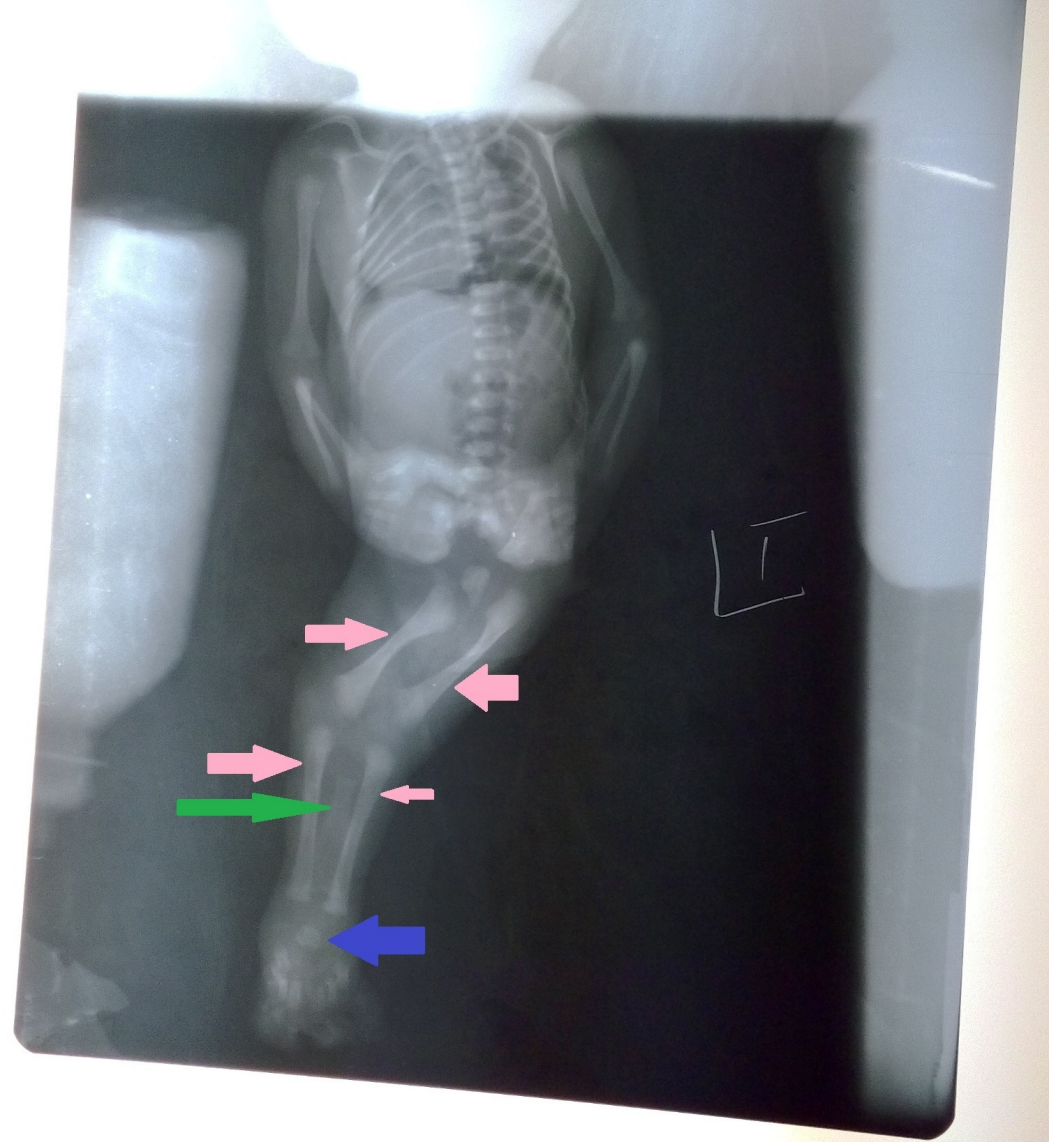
X-ray of newborn with sirenomelia Two different sets of femur and tibia were present (pink arrows); however, a fused fibula (green arrow) and fused talus (blue arrow) were also noted.
